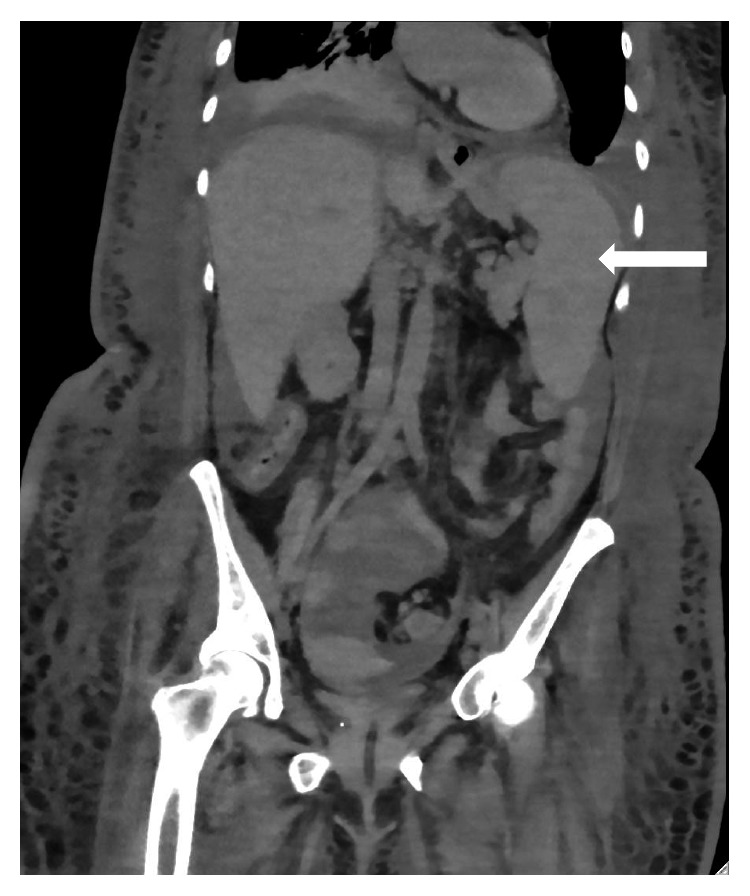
CT scan of the abdomen and pelvis. Abdominal CT scan showing splenomegaly. The spleen was enlarged and was 14 cm in length (white arrow pointing to the enlarged spleen).
